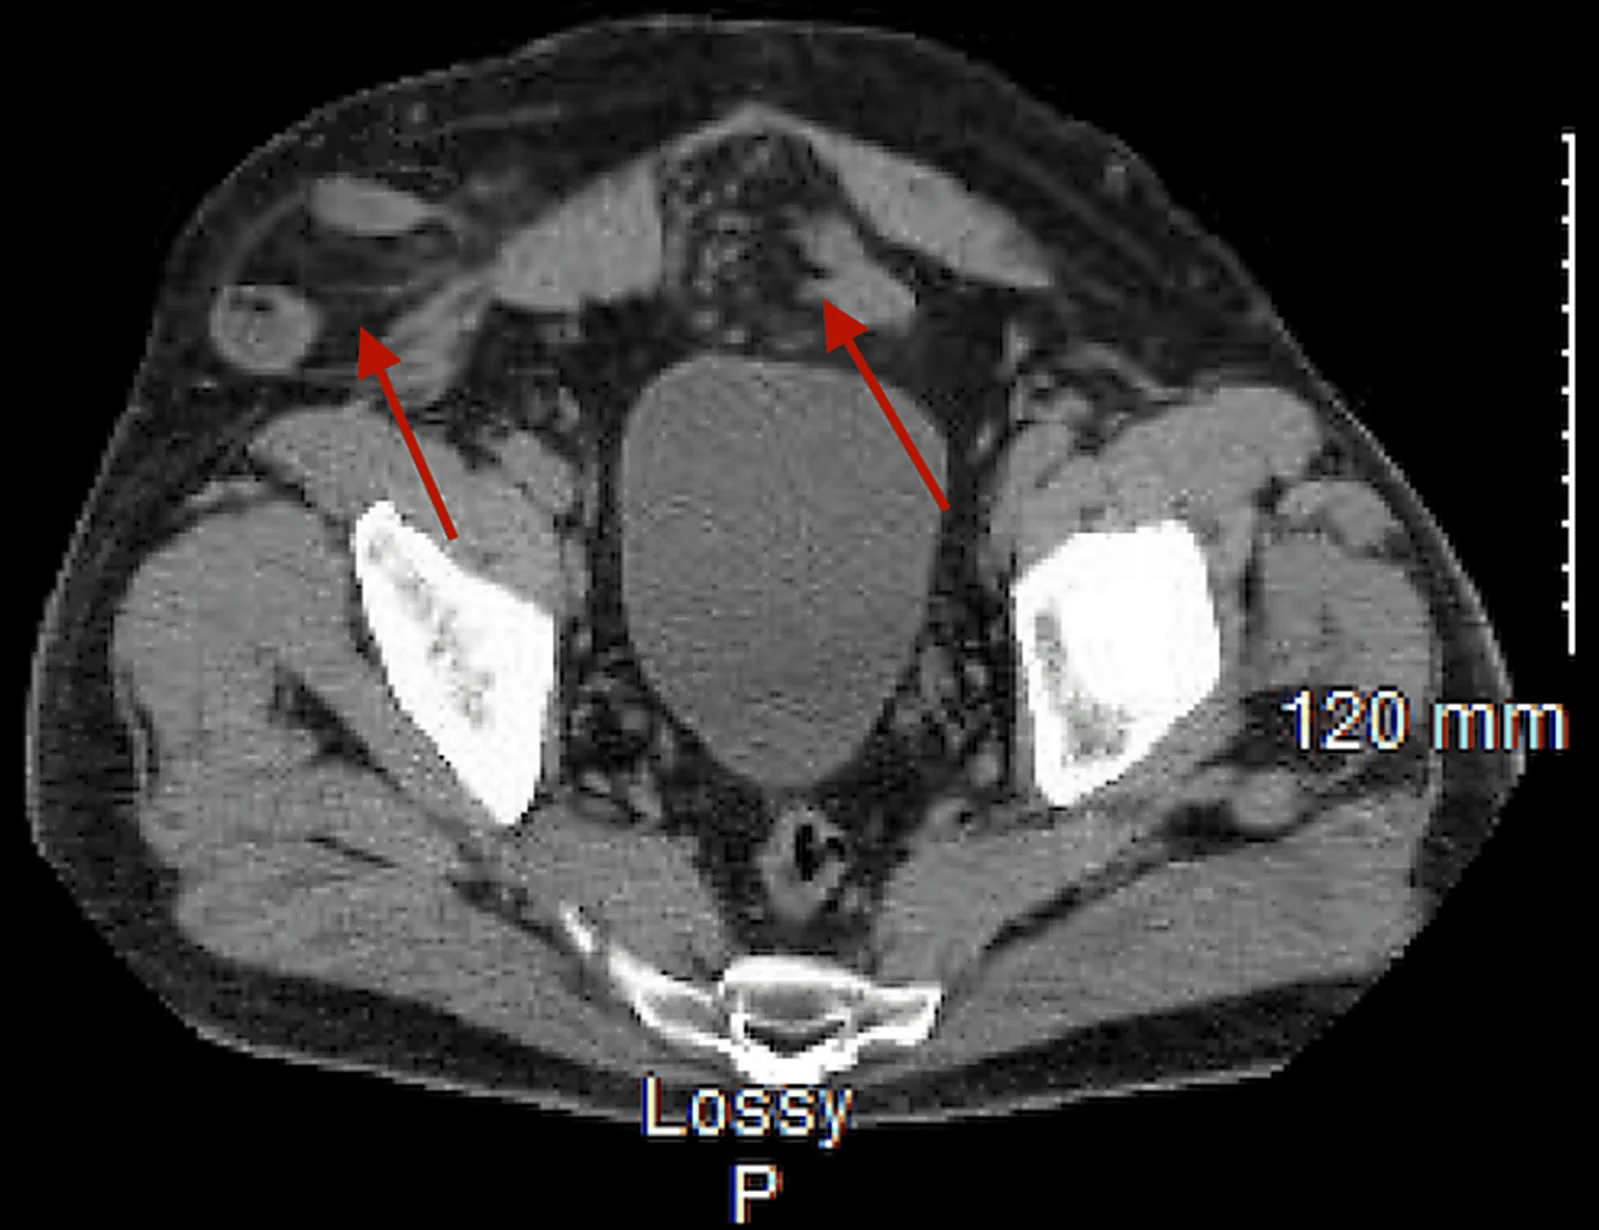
Ventral incisional hernia containing bowel and omentum. Axial CT demonstrates the midline and right paramedian ventral hernia containing small bowel and omentum (red arrows). The fascial defect measured 8.7×10.9 cm, and the hernia sac measured approximately 28.6×16.4×14.8 cm.
